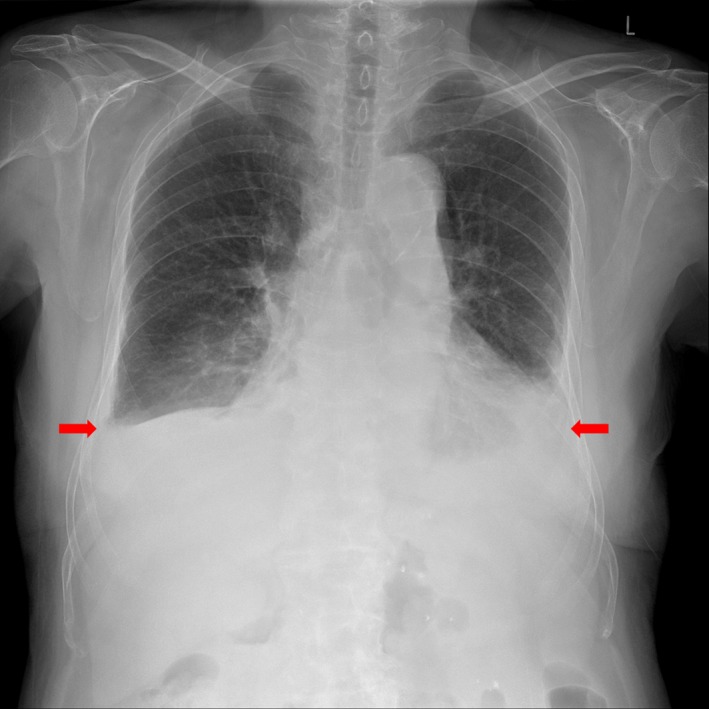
Chest radiograph on admission showing bilateral pleural effusions (arrows)
